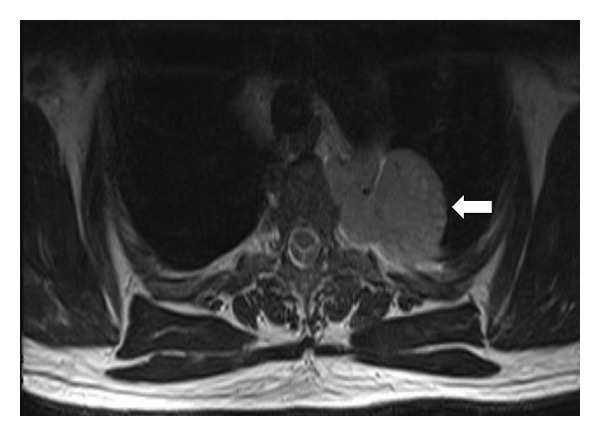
Chest MRI (axial T2 turbo spin echo sequence) in a 50-year-old male patient with Castleman disease. The left paraspinal pleural-based mass is heterogeneously hyperintense to skeletal muscle on T2-weighted sequences (arrow).
